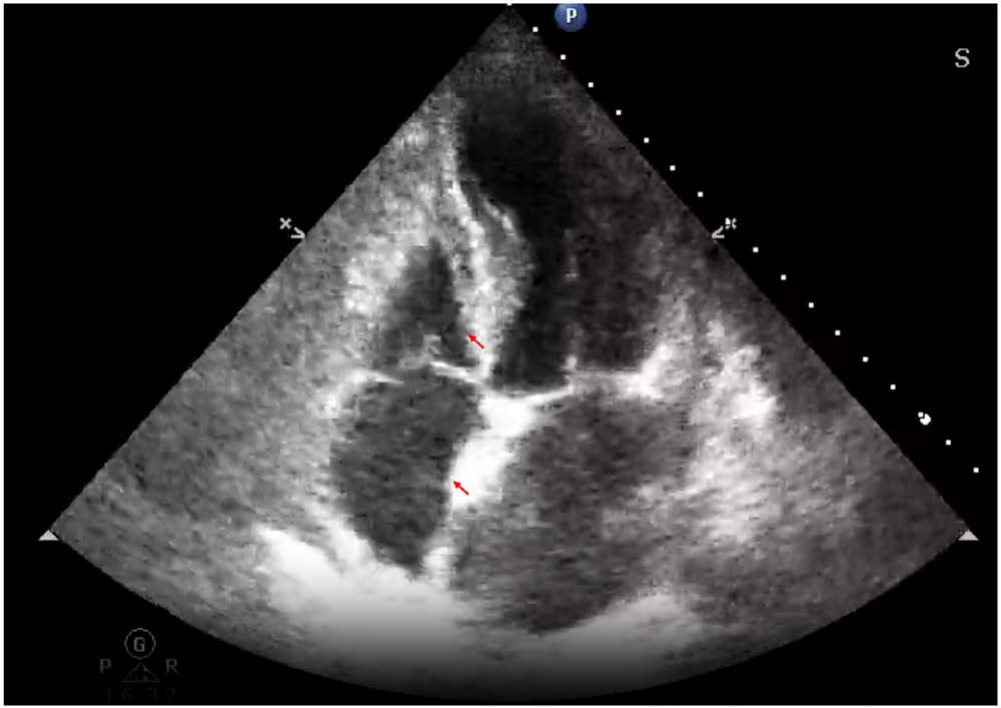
Bedside ultrasound image of transthoracic echocardiography showed that the inner diameter of the right atrium and right ventricle decreased significantly (red arrows).
